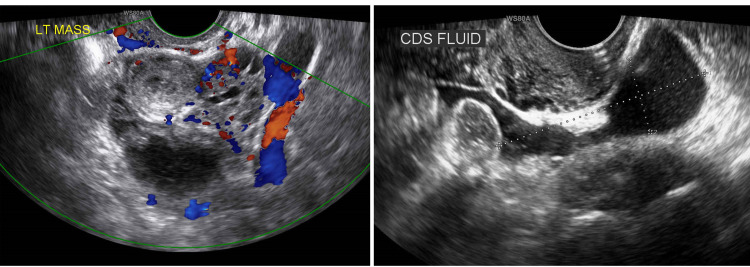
TVUS after one month from the first OPD visit. TVUS two weeks after the third MTX injection. Left tubal ectopic mass with the peripheral flow, measuring 4.9 x 4.3 x 4 cm. Some fluid accumulated in the cul-de-sac, measuring 5 x 1.7 cm. TVUS: transvaginal ultrasonography; OPD: outpatient department; MTX: methotrexate; LT: left; CDS: cul-de-sac.
